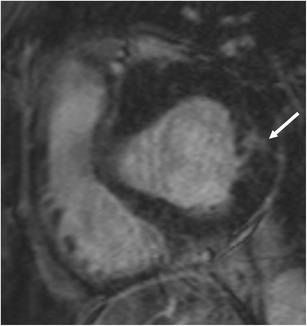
Short-axis post contrast T1-w inversion recovery gradient echo MRI sequence shows patchy mid myocardial LGE in the basal lateral (straight arrow) in a patient with Anderson Fabry’s disease
